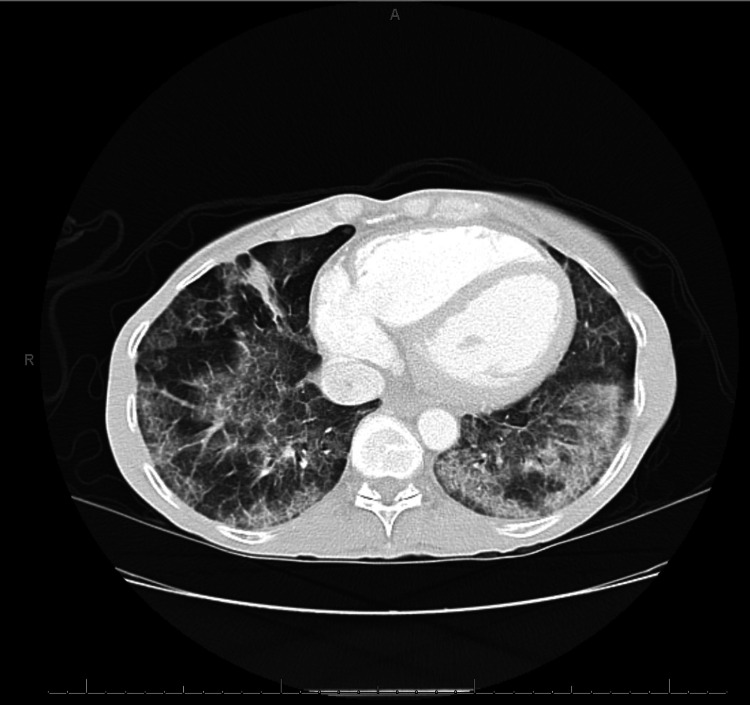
High-resolution axial CT of the chest at the basal level, demonstrating bilateral, predominantly peripheral ground-glass opacities with superimposed interlobular septal thickening (the classic “crazy-paving” pattern) consistent with pulmonary alveolar proteinosis. The diffuse ground-glass opacities and interlobular septal thickening seen on high-resolution computed tomography ("crazy-paving") correlate with her severe exertional dyspnea and reduced diffusing capacity of the lungs for carbon monoxide, reflecting impaired alveolar gas exchange.
